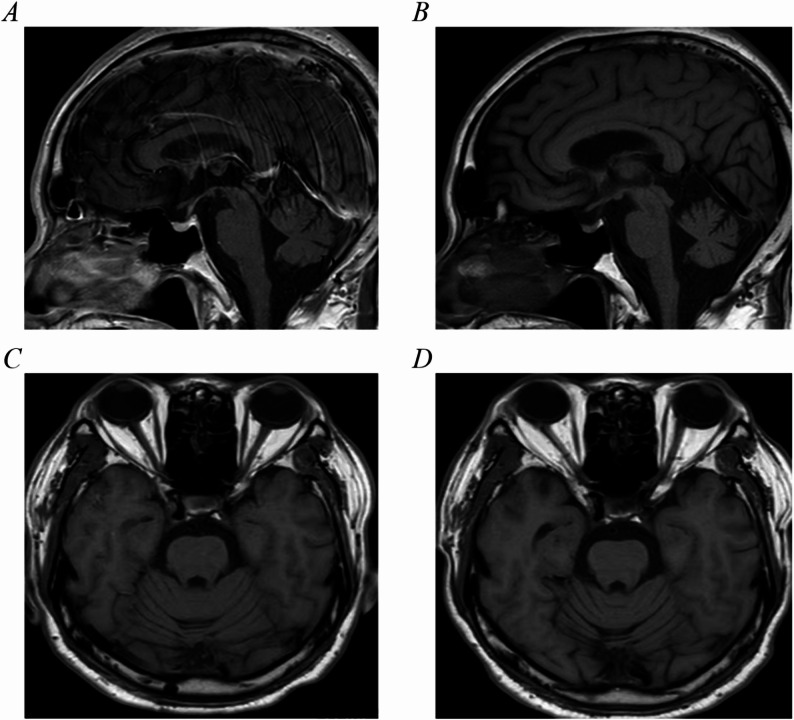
Neuroimaging of Patient 2. A: Sagittal T1-weighted MRI at initial presentation showing no structural abnormalities. B: Sagittal T1-weighted MRI at relapse revealing cerebellar atrophy. C: Axial T1-weighted MRI at initial presentation showing no abnormalities. D: Axial T1-weighted MRI at relapse demonstrating cerebellar atrophy
